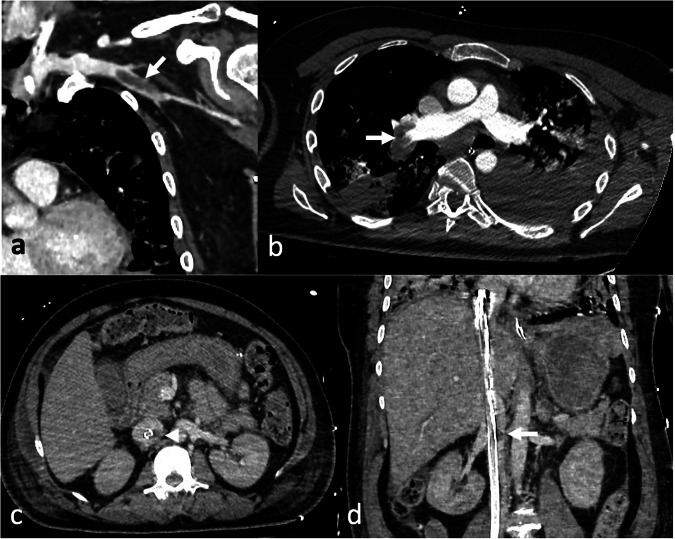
Coronal computed tomography (CT) image in the venous phase (a) depicting a thrombotic filling defect in the left axillary vein (arrow), in a veno-venous (VV) extracorporeal membrane oxygenation (ECMO) supported patient. Axial pulmonary CT angiography in a 51-year-old male with acute respiratory distress syndrome (ARDS) (b), performed after ECMO cannula removal due to malpositioning, reveals an intraluminal filling defect in the right pulmonary artery and right interlobar artery (arrow), indicating central pulmonary embolism. Venous phase axial (c) and coronal (d) CT images in a 34-year-old male with femoro-atrial VV-ECMO demonstrate a focal thrombus attached to the drainage cannula at the left renal vein-inferior vena cava (IVC) junction (arrowhead in c, arrow in d)
